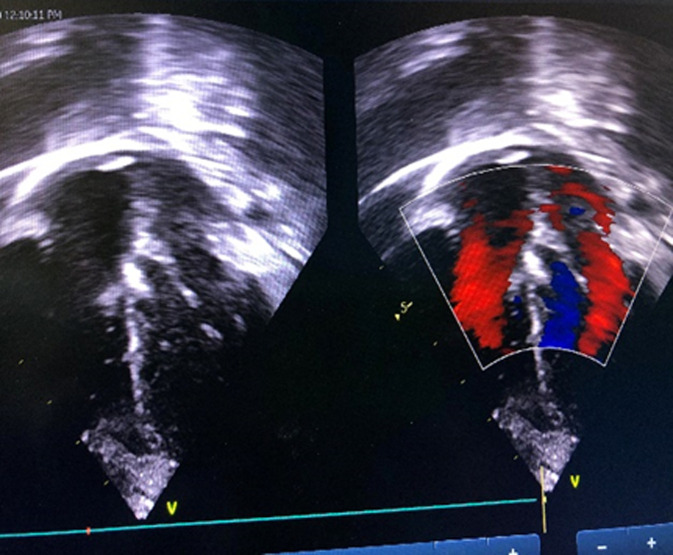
post-procedure echocardiogram
